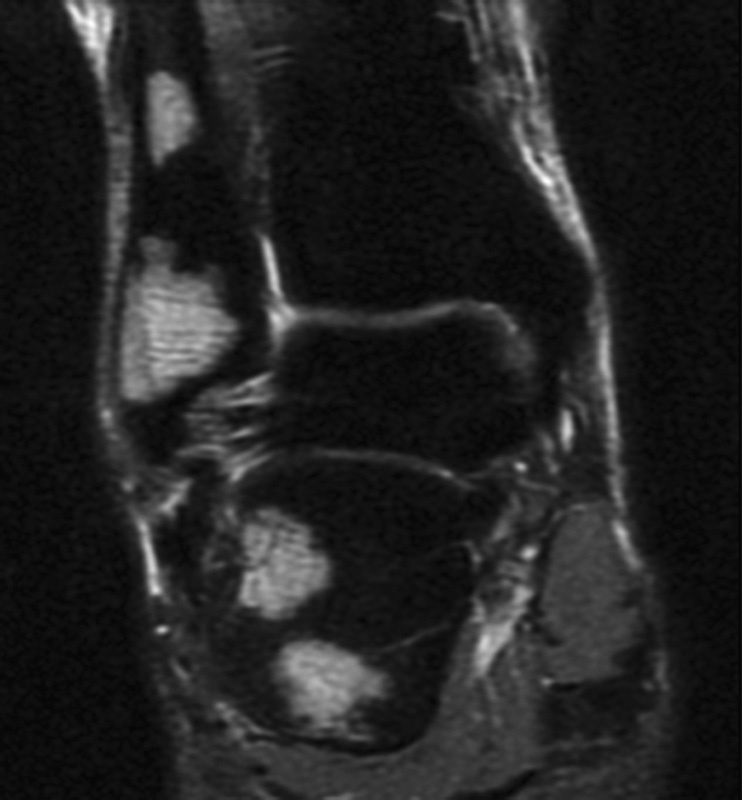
Hyperintense lesions in fibula and calcaneus (T2-weighted magnetic resonance imaging section).
